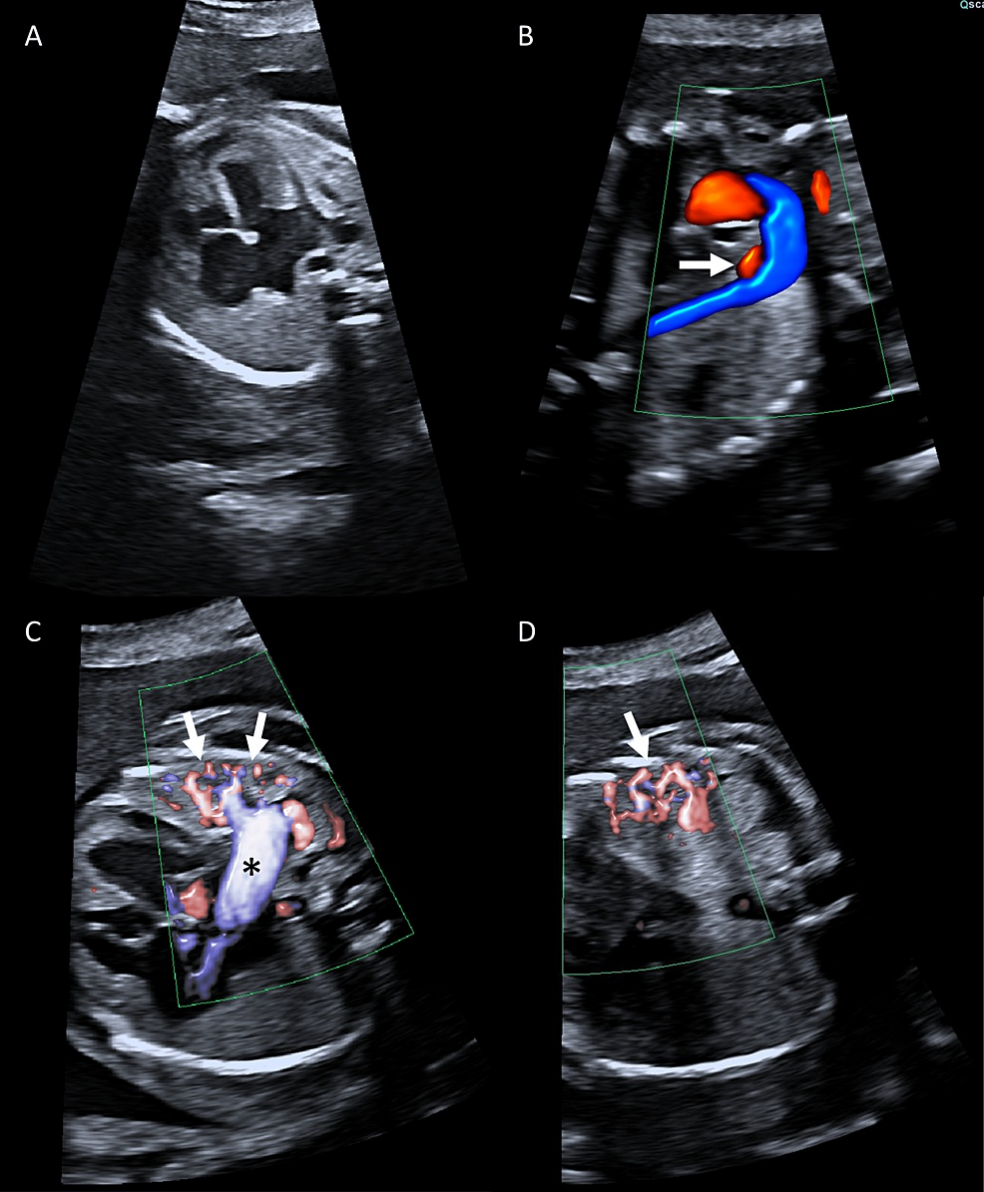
Pulmonary arteriovenous malformations in fetal echocardiogram. Fetal echocardiogram significantly increased cardiothoracic circumference ratio and biventricular hypertrophy (A), left to right shunting through the ductus arteriosus (B, arrow), enlarged left pulmonary vein (C, asterisk), and vascular malformations in the juxtaposed left lung (C and D, arrows).
